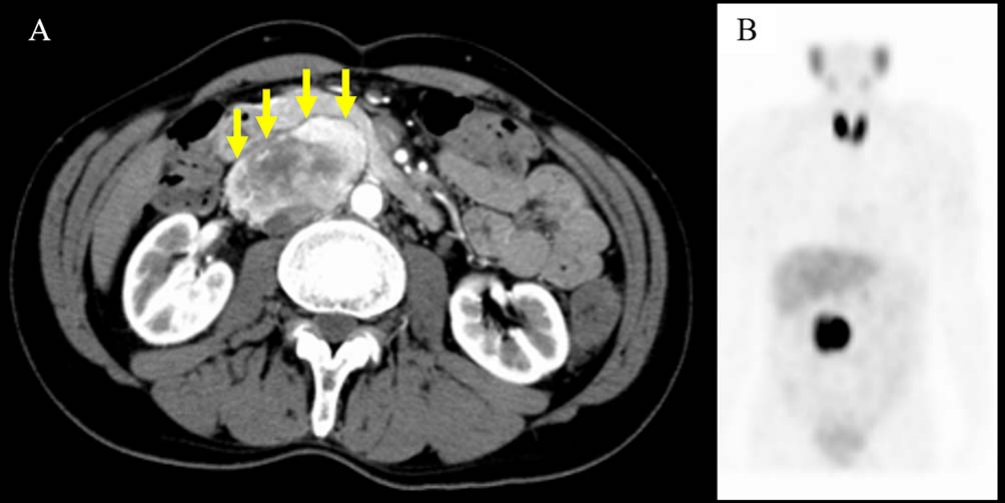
Computed tomography scan and metaiodobenzylguanidine scintigraphy. (A) Imaging findings showing a well-defined mass with heterogeneous enhancement located adjacent to the pancreatic head. A fat plane was identified between the mass and pancreas (arrows). (B) Metaiodobenzylguanidine scintigraphy revealed accumulation at the same site of the abdominal tumor.
